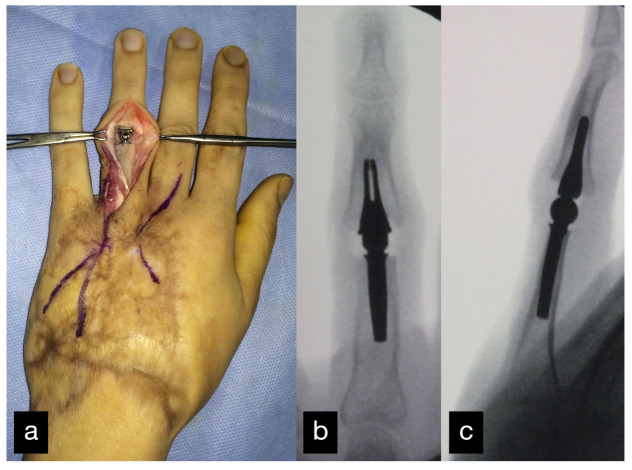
Replacement of the PIP joint of the left ring finger with a joint prosthesis. Intraoperative picture (a) and postoperative X-ray a.p. (b) and sideways (c).
